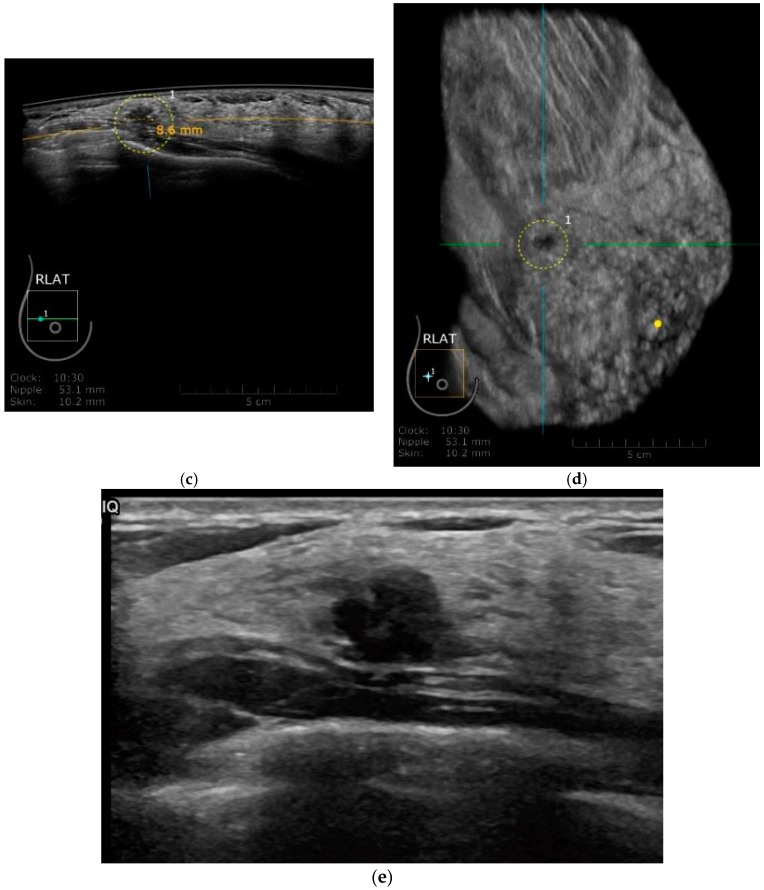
(a,b) CC and MLO digital mammograms in a 56 year old woman with heterogenously dense breasts with an occult breast cancer. Automated whole breast ultrasound (ABUS). (c) transverse image of ABUS and (d) reconstructed coronal image demonstrate an irregular hypoehoic mass. (e) Handheld ultrasound confirms a 0.8 cm irregular, heterogenous hypoechoic mass. Pathology demonstrated mixed invasive ductal and invasive lobular carcinoma, grade 2, ER positive, PR and HER2 negative.
